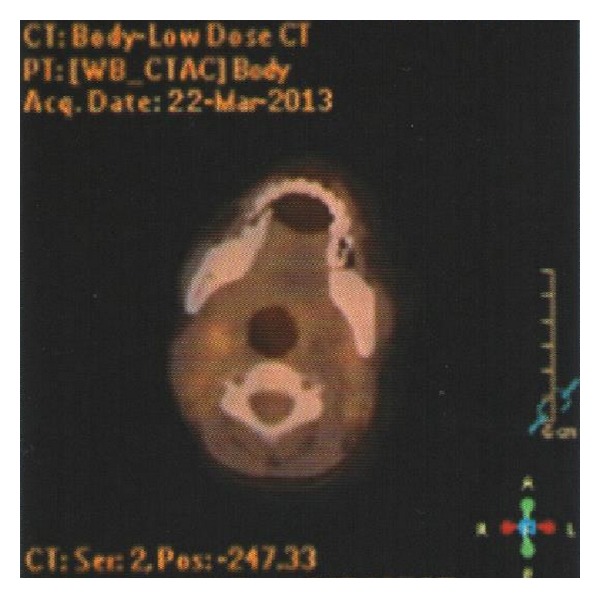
FDG-PET/CT, cervical lymph nodes.
